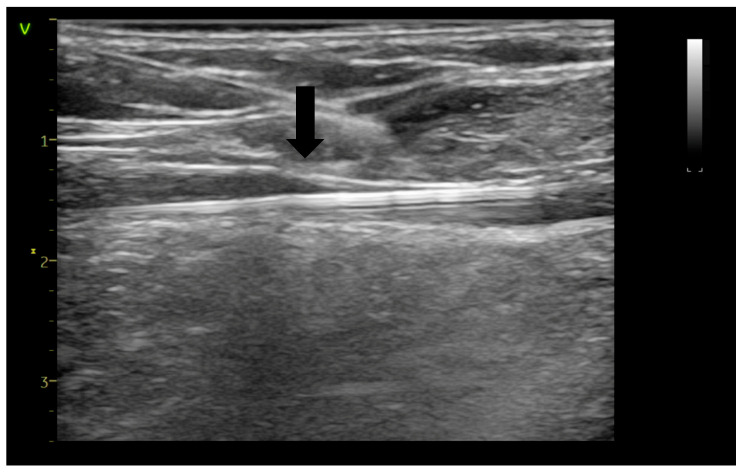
Ultrasound-guided tumescent anesthesia applied in the saphenous compartment (arrow—anesthesia needle tip).
